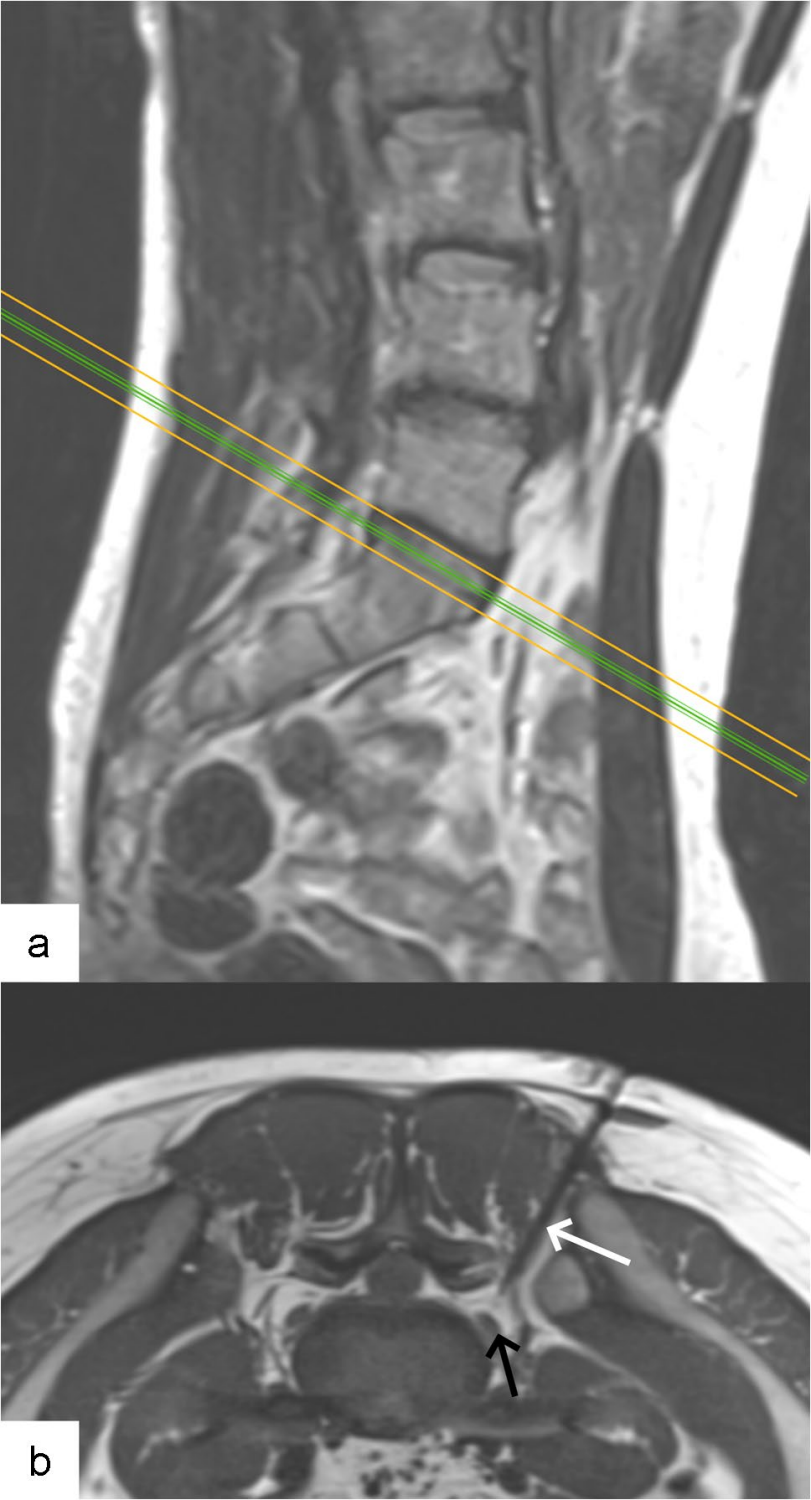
Non-contrast MRI scan obtained in nerve root infiltration in 24-year-old man. a Sagittal T2 turbo spin echo-weighted image showing oblique cranioventral access plane (green line) chosen to access L5 nerve within L5-S1 neural foramen. b Axial oblique proton density-weighted image showing 20G needle (white arrow) arriving behind left L5 nerve (black arrow)
